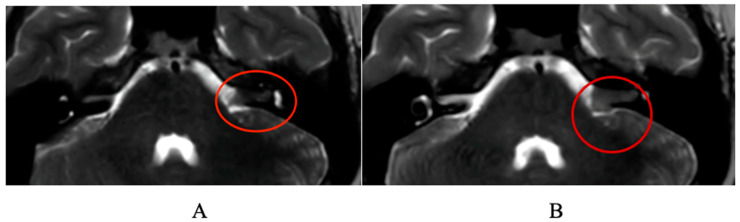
Comparison of the two MRI-obtained scans showing the increase in the lesion (highlighted by a red circle) from 13.8 × 5 × 6 mm (A) to 17 × 5 × 10 mm (B).
